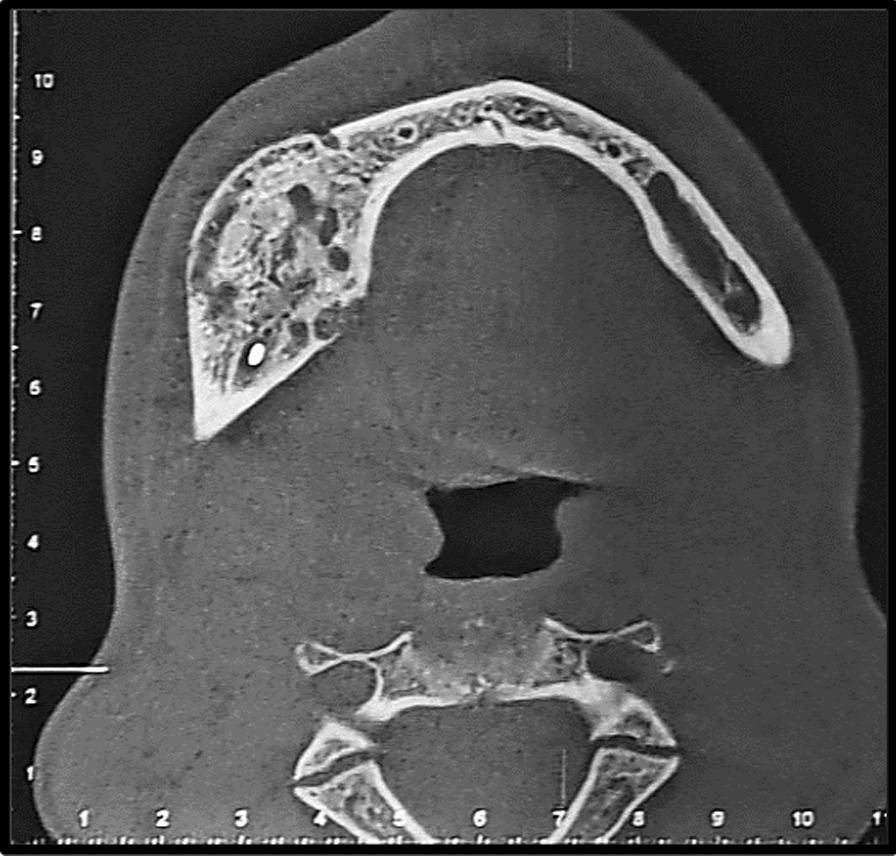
Cone bean computed tomography image (axial view) revealed expansion, thinning and loss of continuity of both buccal and lingual cortices
